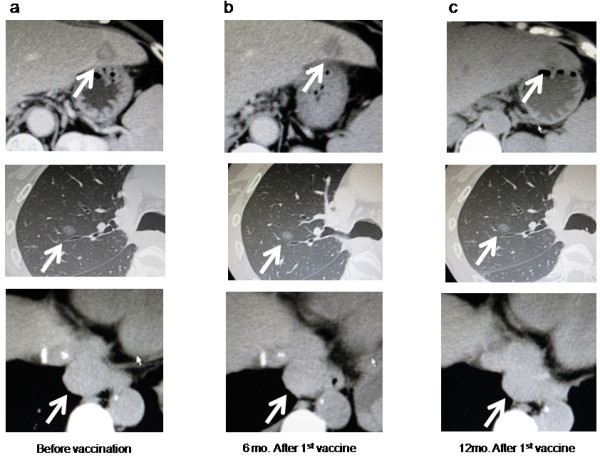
Clinical response assessment in the longest-surviving patient, patient 4. CT imaging of the liver, lung and para-aortic lymph node metastasis before vaccination (a), 6 months after 1st vaccination (b) and 12 months after 1st vaccination (c). The tumor sizes were stable across the 505 days after 1st vaccination.
